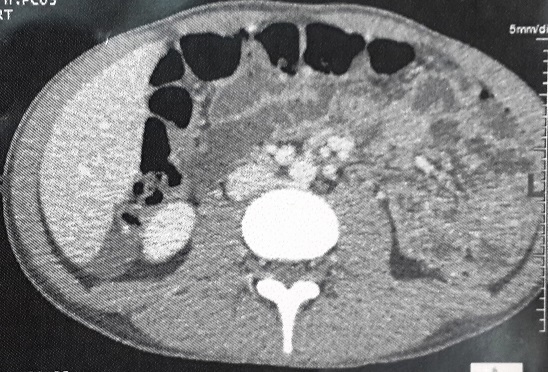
Computed tomography scan revealed a circumferential thickening of the terminal ileum with luminal stenosis and dilated intestine upstream: mesenteric lymphadenopathies were also noted
